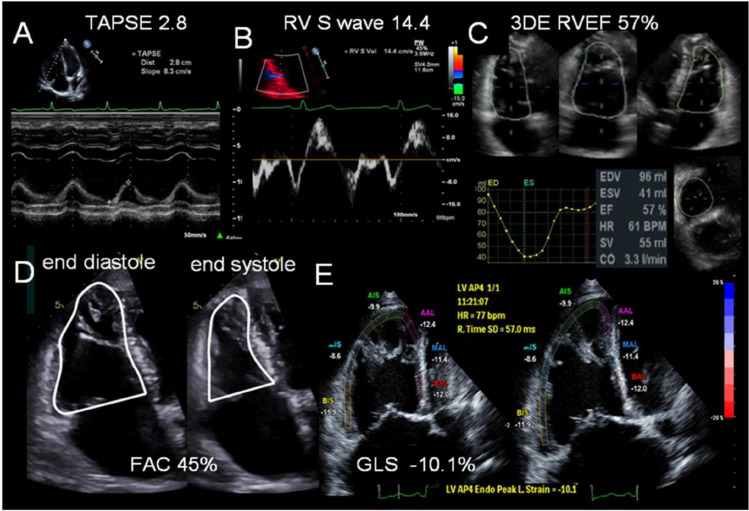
Echocardiographic parameters for the quantitative assessment of RV function. A Tricuspid annular plane systolic excursion (TAPSE), B right ventricular systolic wave velocity measured with Tissue Doppler Imaging (RV S′), C right ventricular ejection fraction measured by 3D-echocardiography (3DE RVEF), D fractional area change (FAC), E 2D right ventricular global longitudinal strain (GLS). *Original image republished without need for permission under a Creative Commons Attribution-Non-Commercial-No Derivatives 4.0 International License from Rana et al.* [37]
